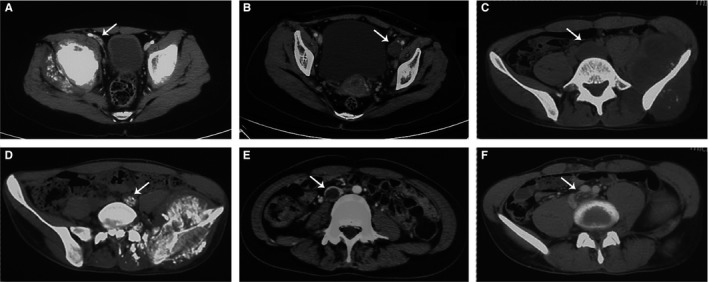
Manifestations of venous tumor thrombus (VTT) on plain and enhanced CT scan. A, A VTT located at the right external iliac vein (arrow) showed unchanged caliber of the vessel compared with the contralateral side. B, A VTT located at the left external iliac vein (arrow) showed enlarged caliber of the vessel compared with the contralateral side. C, A VTT located at the left common iliac vein (arrow) showed low density compared with the muscles on plain CT scan. D, A VTT located at the left common iliac vein (arrow) showed apparent calcification within the vascular lumen on plain CT scan. E, A VTT located at the inferior vena cava (arrow) showed filling defect within the vascular lumen on contrast enhancement. F, A VTT located at the left common iliac vein (arrow) showed streak‐like enhancement within the filling defect on contrast enhancement
